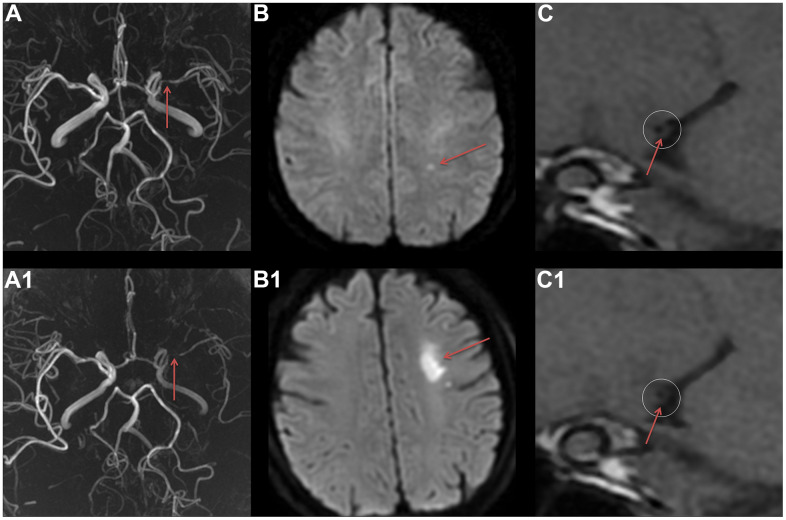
The VWMRI of MCA plaque characteristic in a patient with ischemic stroke recurrence. A female patient, 56 years old, experienced her first ischemic stroke in August 2019. MRA revealed severe stenosis of the M1 segment of the left MCA (A). At the same time, DWI displayed a punctate high signal in the left centrum semiovale (B), and VWMRI revealed centripetal thickening of the MCA and severe lumen stenosis (C). In November 2019, the patient experienced a recurrent ischemic stroke. MRA displayed an aggravated stenosis of the M1 segment of the left MCA (A1), and DWI showed a new patchy high signal in the left centrum semiovale (B1). Furthermore, VWMRI revealed an aggravated centripetal thickening of the MCA and a worsening lumen stenosis (C1).
